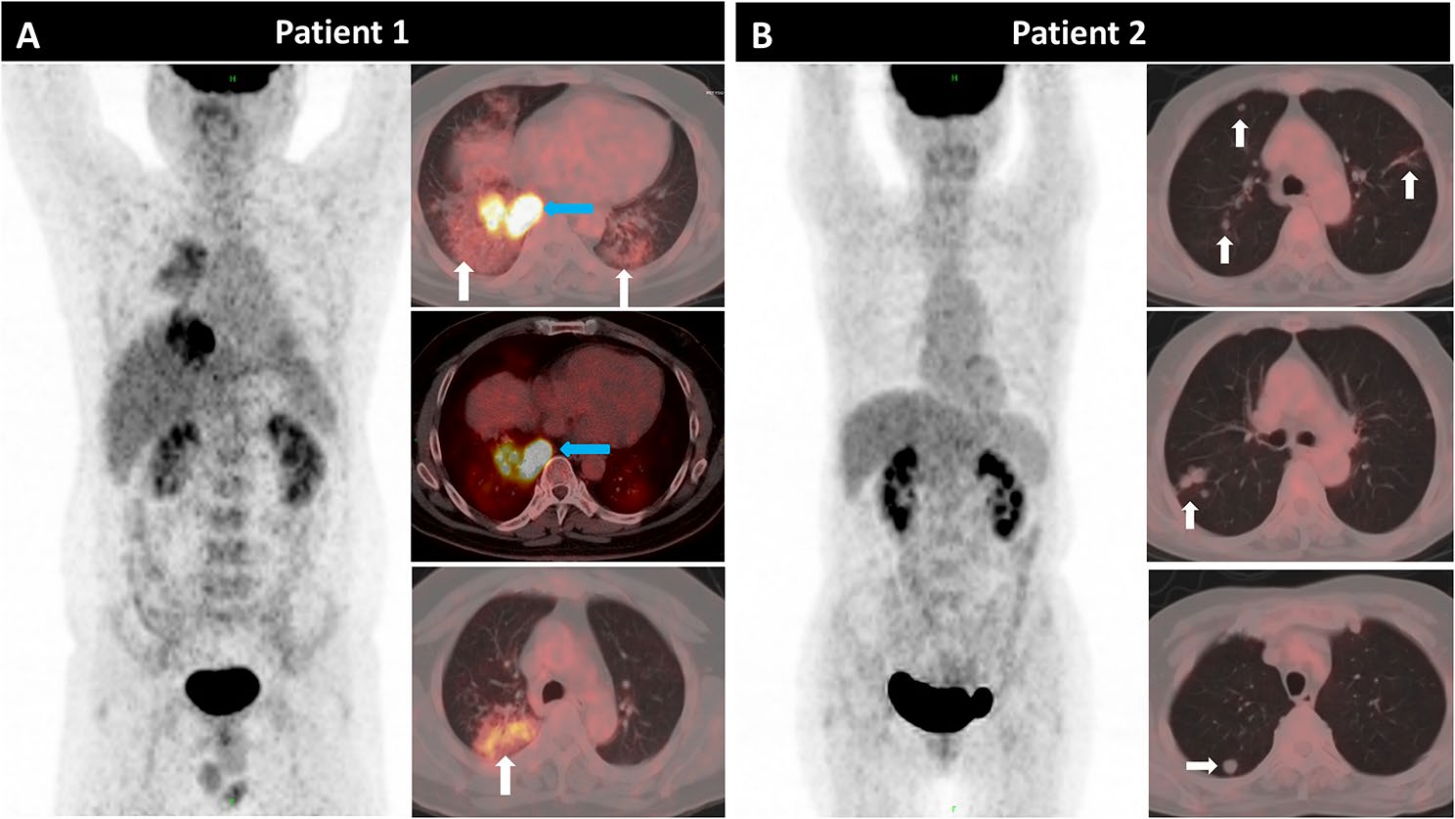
Patient 1 (No.1): A 38-year-old male patient presented with cough, phlegm and intermittent fever for two months. The pathology of the pulmonary puncture suggested chronic granulomatous inflammation of the lungs. Finally, metagenomics next-generation sequencing (mNGS) of alveolar lavage fluid confirmed *M. abscessus* infection. Multiple patchy shadows (white arrows, SUV_max_ = 11.3) and consolidation (blue arrows, SUV_max_ = 3.7) in both lungs on the axial slices of ^18^F-FDG PET/CT scan images.Patient 2 (No.10): A 60-year-old female patient with a history of acute lymphocyte leukemia. After one cycle of chemotherapy, opportunistic intracellular mycobacterial infection appeared in both lungs and was confirmed by TB-DNA-PCR of the sputum. The patient was given active anti-infection treatment (oral azithromycin + ethambutol + rifampicin for 5 months). To assess the tumor activity after treatment, an ^18^F-FDG PET/CT scan was performed, which revealed multiple FDG-unavid nodules (white arrows, SUV_max_ = 2.2) in the right lung. The pathology of the wedge resection of the right lung nodule indicated pulmonary granulomatosis
